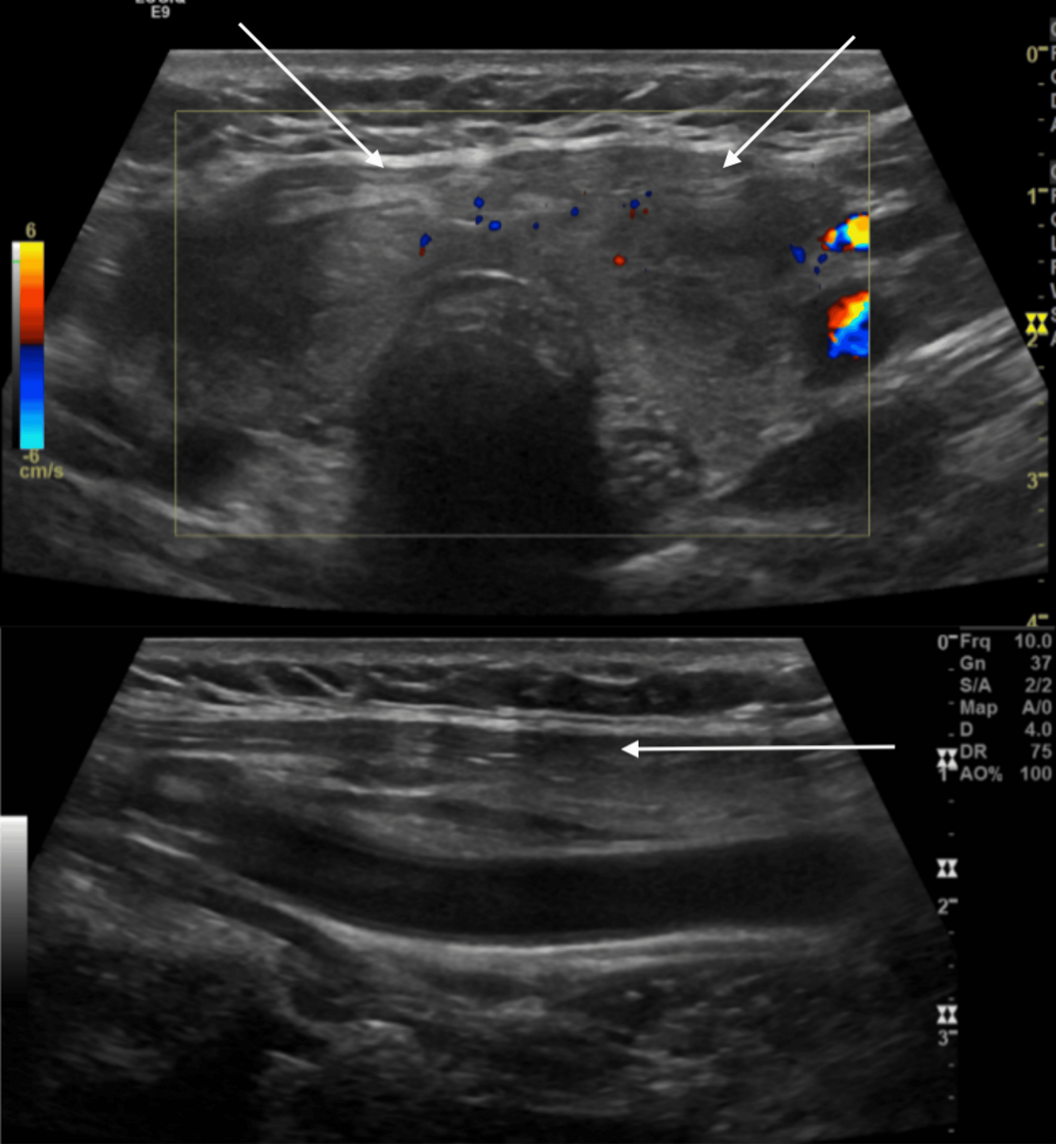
Thyroid ultrasound indicated an enlarged thyroid gland showing heterogeneous echotexture with normal vascularity.
